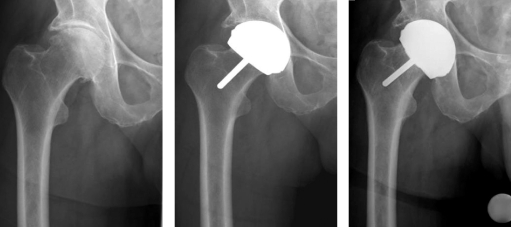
Radiographs of case 2, a male patient with osteoarthritis in the right hip. A. A preoperative anteroposterior radiograph showing good bone quality. B. A postoperative anteroposterior radiograph showing that the implant is well-positioned. C. 7 months postoperatively, the patient developed pain in his hip always after intensive physical activity. Radiographs showed that the femoral component had now turned to a varus position.
